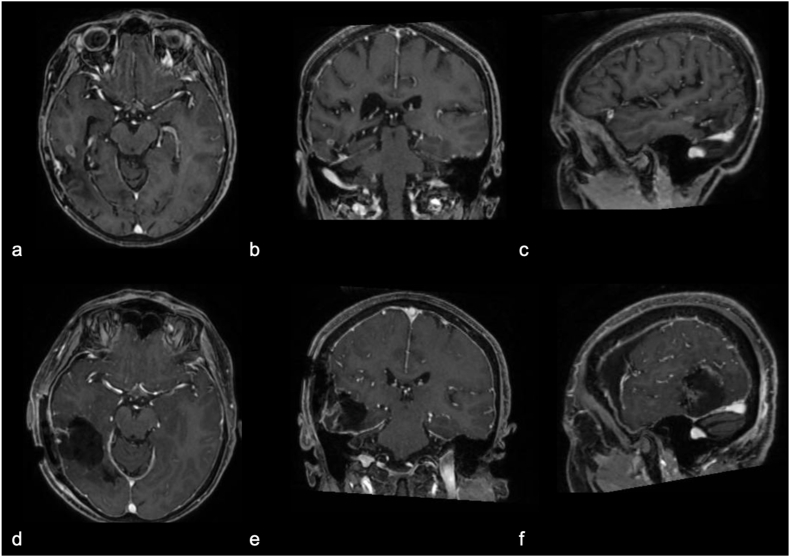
Preoperative brain MRI images, T1-weighted sequences with gadolinium, showing (a) Axial, (b) Coronal, and (c) Sagittal views of the recurrence of a right temporal glioblastoma; postoperative images (d, e, f) after complete tumor resection.
